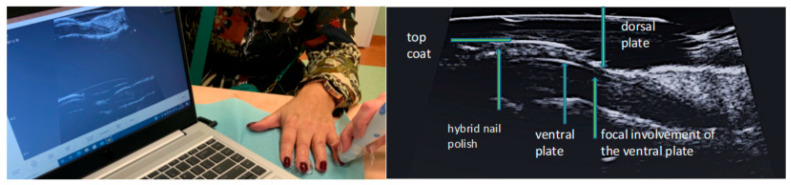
Ultrasound examination of nails with hybrid polish.
